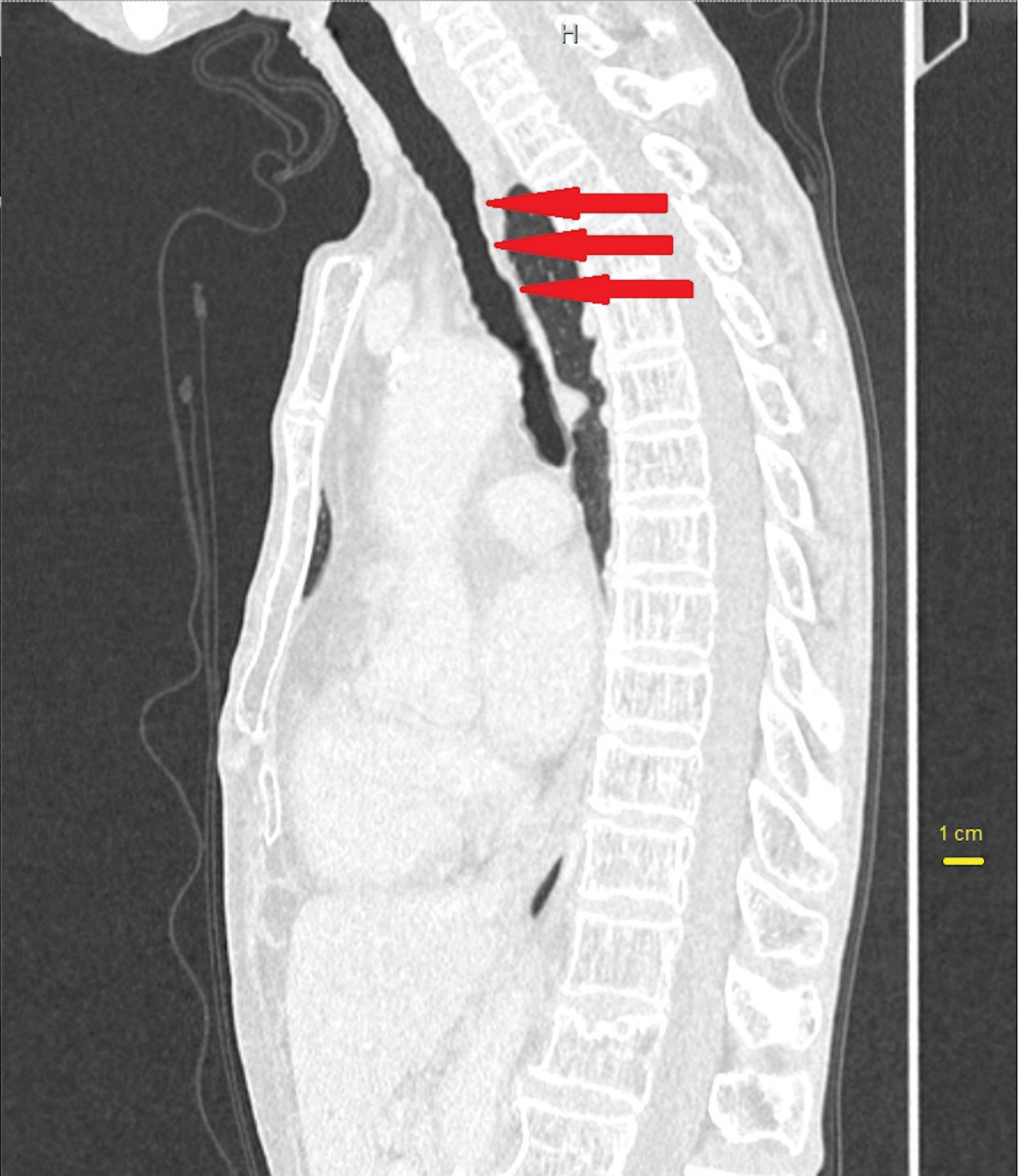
Chest CT image before surgery in sagittal section. Red arrows indicate tracheal stenosis. CT revealed the stenosis is located 5 cm distal to the glottis and the narrowest inner diameter of the stenosis is approximately 10 mm.
